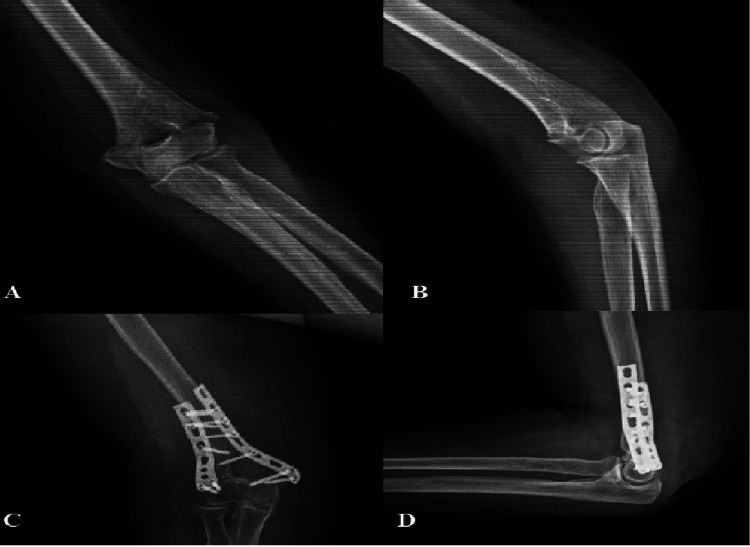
80-year-old female with a low transcondylar fracture (A) Preoperative anteroposterior and (B) lateral radiographs of a low transcondylar fracture (80-year-old female). (C, D) Postoperative anteroposterior and lateral images at four months; the union was achieved successfully
